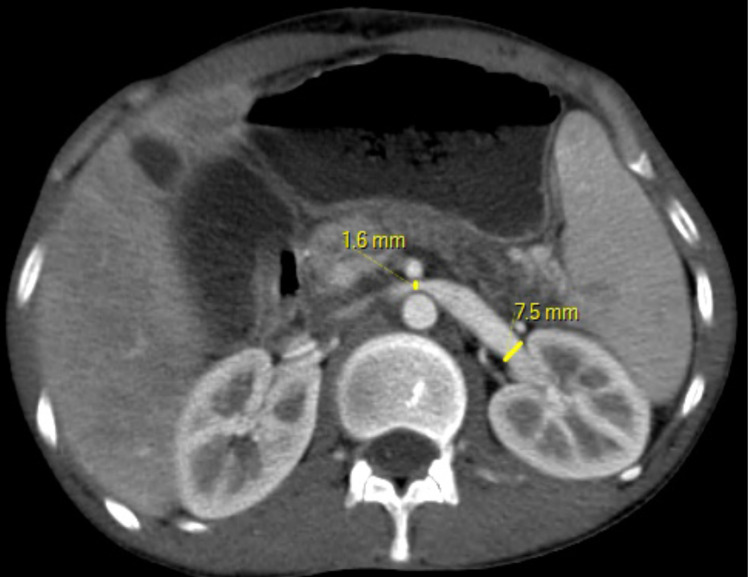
Abdominopelvic CT of a 30-year-old male patient presented with left chest pain and for work up for unknown primary. Post contrast axial abdominal CT at the level of renal vein shows compression of left renal vein as it passes between abdominal aorta and superior mesenteric artery (SMA).
